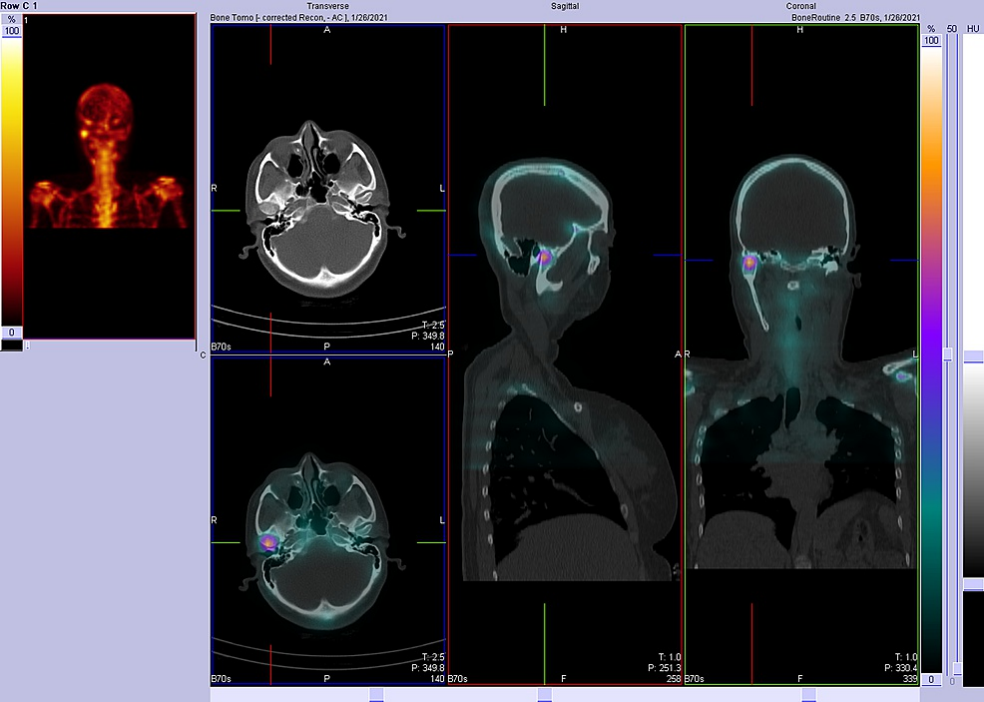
SPECT image demonstrating intense focal activity that corresponds to the right mandibular condyle which appears enlarged. It is associated with elongation of the right mandibular neck and tilt of the mandible to the left side. SPECT: single-photon emission computed tomography
